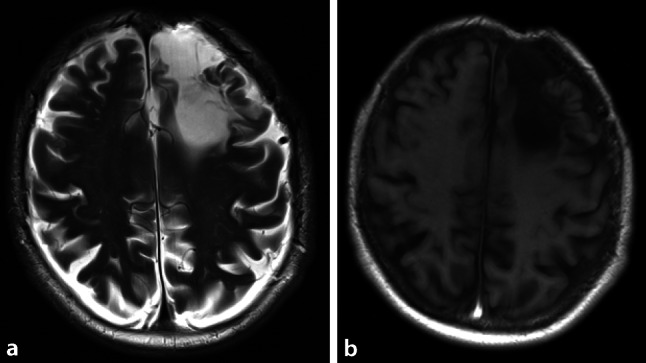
Secondary posttraumatic arachnoid cyst below the osseous defect of the left frontal bone with subsequent dilatation of the left lateral ventricle on cranial magnetic resonance imaging (a T2-weighted, b T1-weighted)
